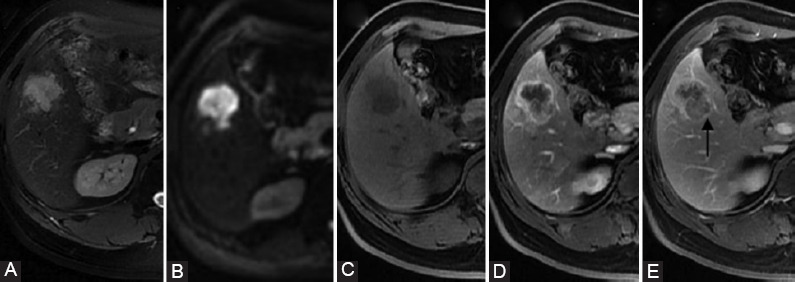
LR-M intrahepatic cholangiocarcinoma (ICCA). A surgically confirmed ICCA in a 46-year-old male with hepatitis B virus infection. (A) T2WI-FS image, (B) diffusion-weighted imaging (DWI) image (b = 1000 sec/mm), (C) precontrast image, (D) late arterial, and (E) delayed phase images. The tumor showed target appearance on DWI image. Rim arterial phase hyperenhancement during the arterial phase, progressive central enhancement during the delayed phase, and lobulated capsule appearance (arrow).
